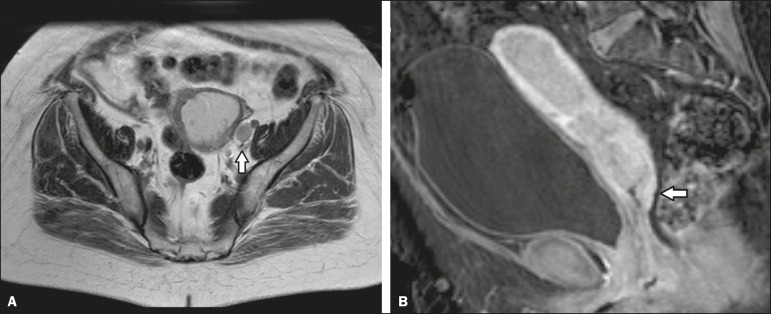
Axial T2-weighted image (A) and gadolinium-enhanced
